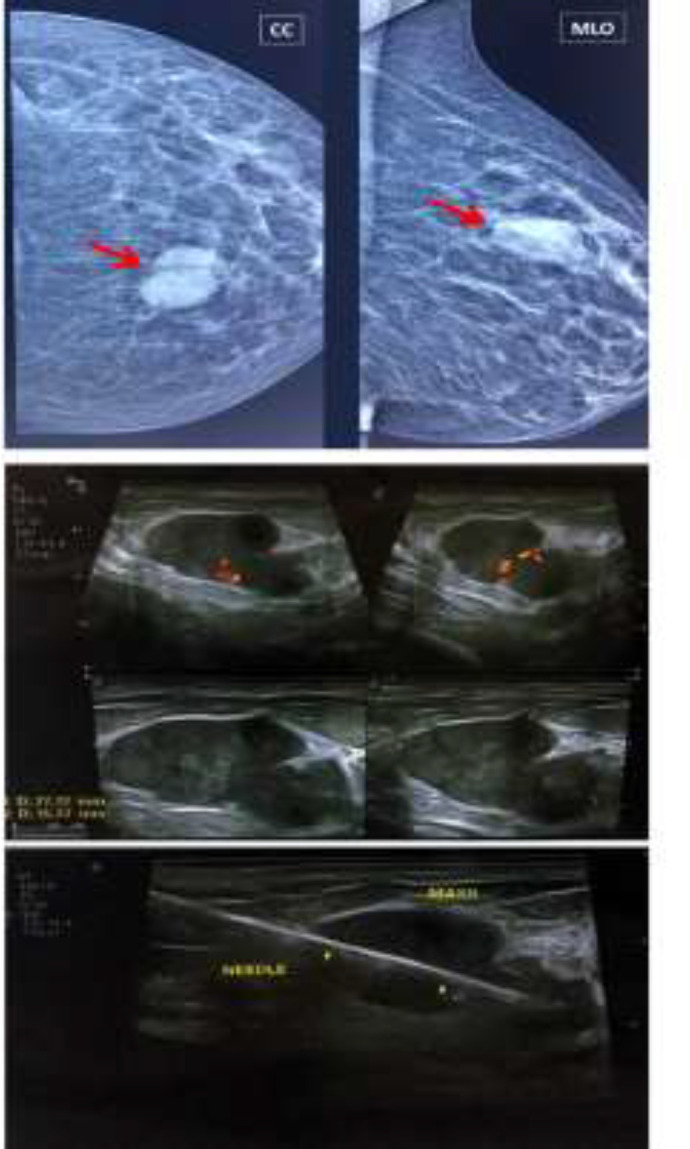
Mammography, ultrasound, and ultrasound-guided core needle biopsy from a left breast mass (BI-RADS 3), which was proved by pathology to be a fibroadenoma
